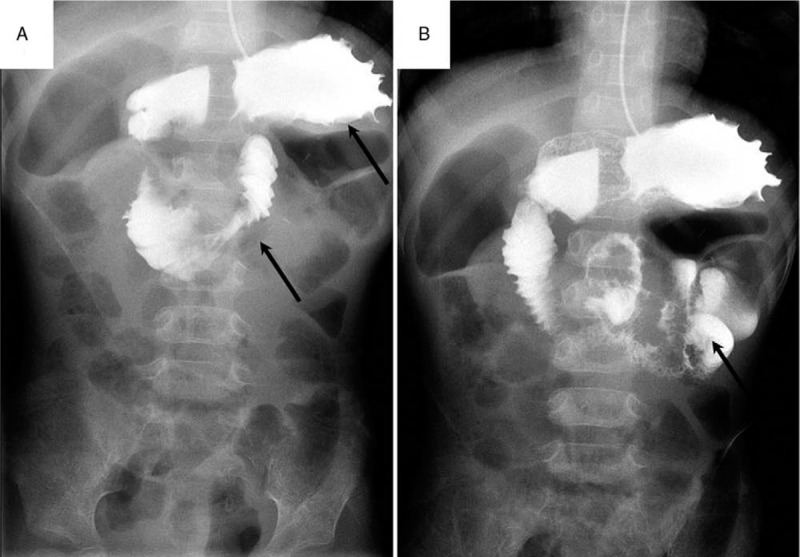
Gastrointestinal barium meal radiography demonstrates an unsmooth outline of fundus of stomach, a stricture between the duodenum and proximal jejunum (arrow, A), and featheriness or spring-like structure cannot be observed in jejunum (arrow, B).
